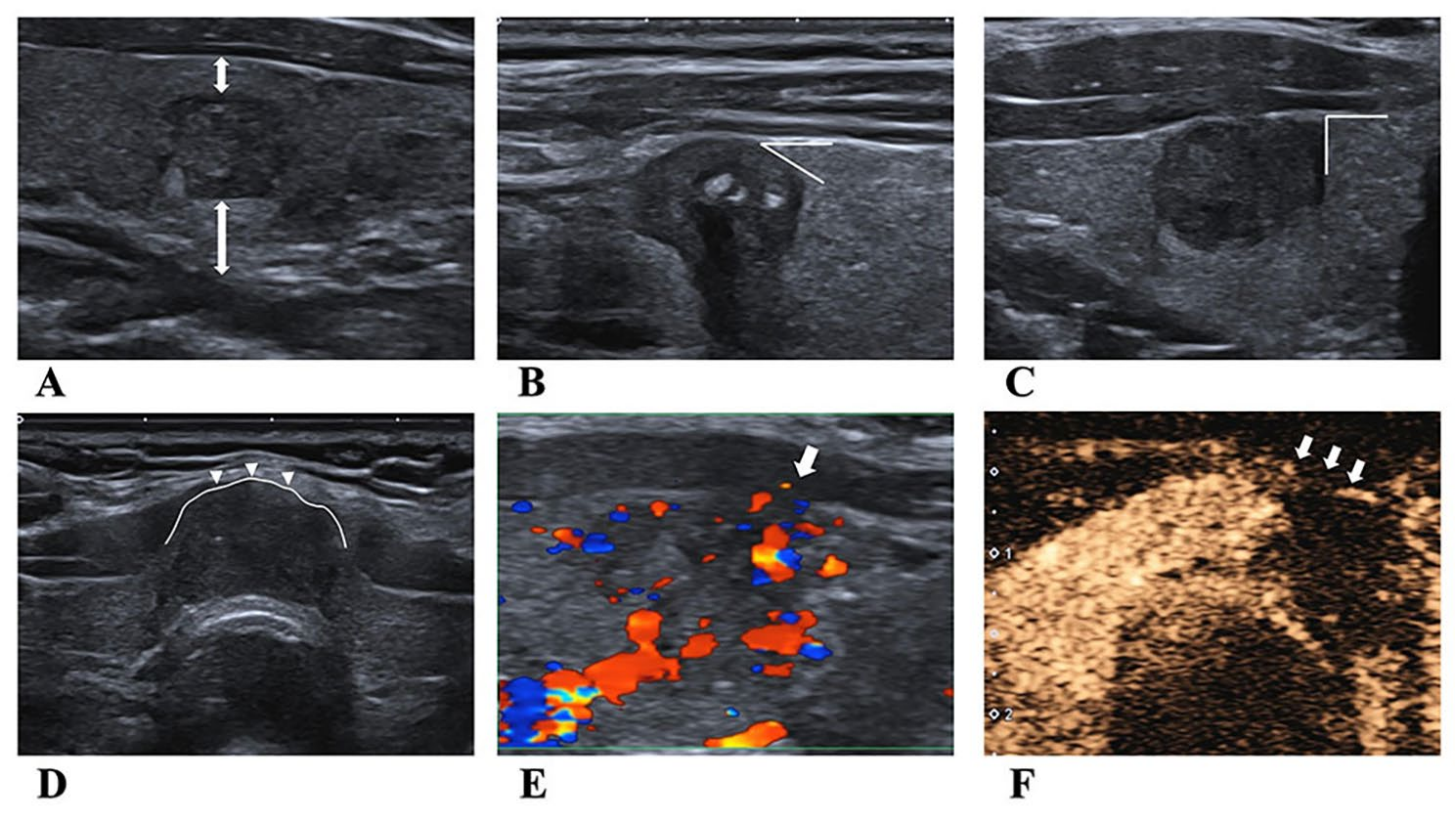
Ultrasonography for diagnosis of extrathyroidal extension (A) The lesion is not in contact with the capsule. According to the classification criteria, the contact angle with the thyroid capsule is 0°. (B) The lesion formed an acute angle with the thyroid capsule. (C) The lesion formed a straight angle with the thyroid capsule. (D) Thyroid capsule deformation for the bulging nodule. (E) The arrow indicates that the CDFI vascularity of suspicious extrathyroidal extension nodules extended beyond the thyroid capsule. (F) The arrows pointing at contrast-enhanced ultrasound imaging showed discontinuous enhancement of the anterior thyroid capsule
